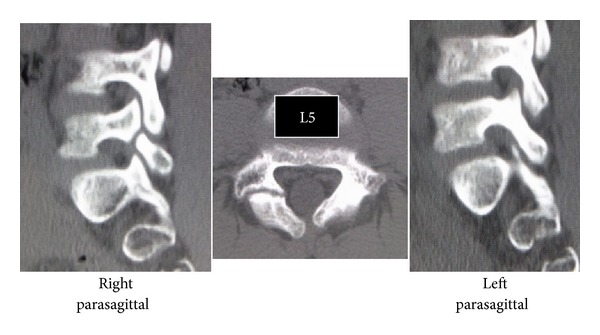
CT of Case 2. The right side unilateral L5 spondylolysis is considered to be at the terminal stage.
